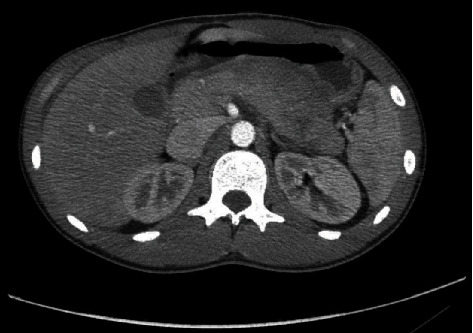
CT scan at presentation in emergency room.
